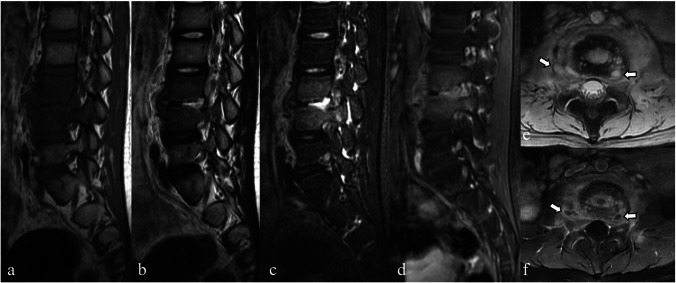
Magnetic resonance images of a 9-year-old female patient with infectious spondylitis. Sagittal T1-weighted (a), T2-weighted (b), short tau inversion recovery (STIR) (c), and fat-suppressed contrast-enhanced T1-weighted (d) images demonstrate destroyed L3–L4 intervertebral discs, characterized by disc space narrowing, hyperintense T2-weighted/STIR signals, and enhancement. Despite edematous marrow of the surrounding L3 and L4 vertebral bodies seen as hypointense areas on the T1-weighted image and hyperintense areas on the T2-weighted/STIR images with mild diffuse enhancement, no significant bone destruction or collapse are noted. Axial T2-weighted (e) and fat-suppressed contrast-enhanced T1-weighted (f) images show prominently thickened soft tissue in the entire perivertebral region and small abscesses within the intervertebral disc and right para-vertebral region (arrows)
